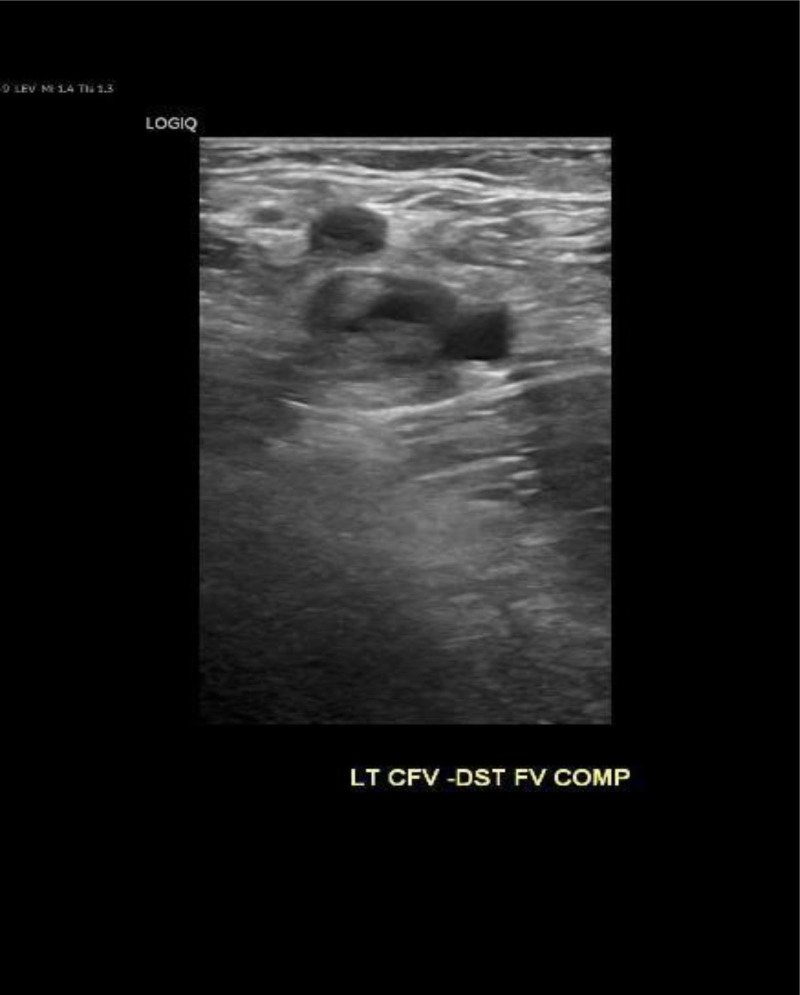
Duplex ultrasound showing DVT. DVT = deep vein thrombosis.
